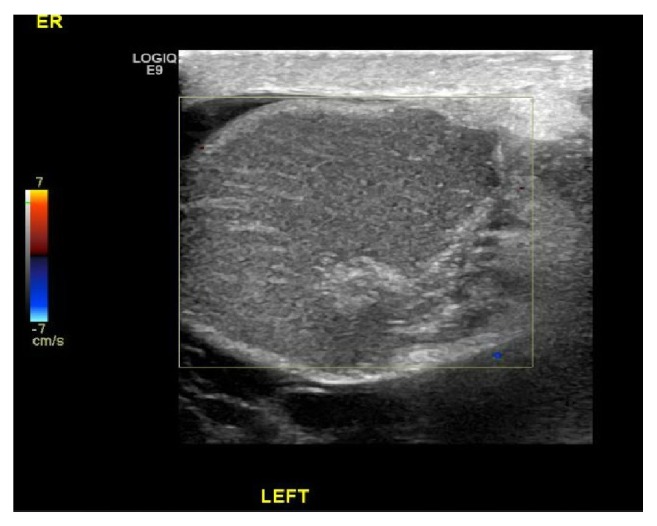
Scrotal ultrasonography showed absent testis blood flow.
